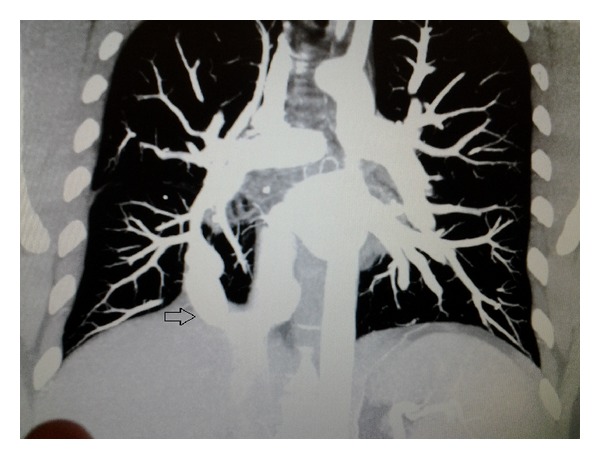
Coronal plane CTA showing anomalous vein drainage into suprahepatic IVC.
